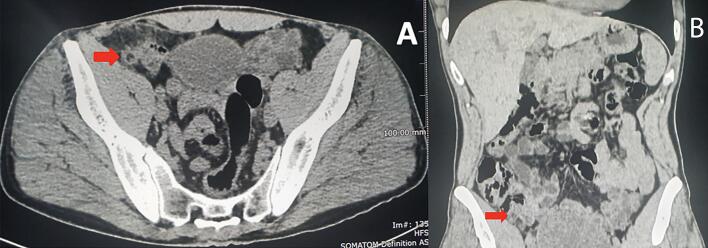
A,B. Abdominal CT showing a dilated appendix with thickened walls and a thin-walled 5 mm appendiceal diverticulum (red arrow). A transverse view, B coronal view. (For interpretation of the references to colour in this figure legend, the reader is referred to the web version of this article.)
